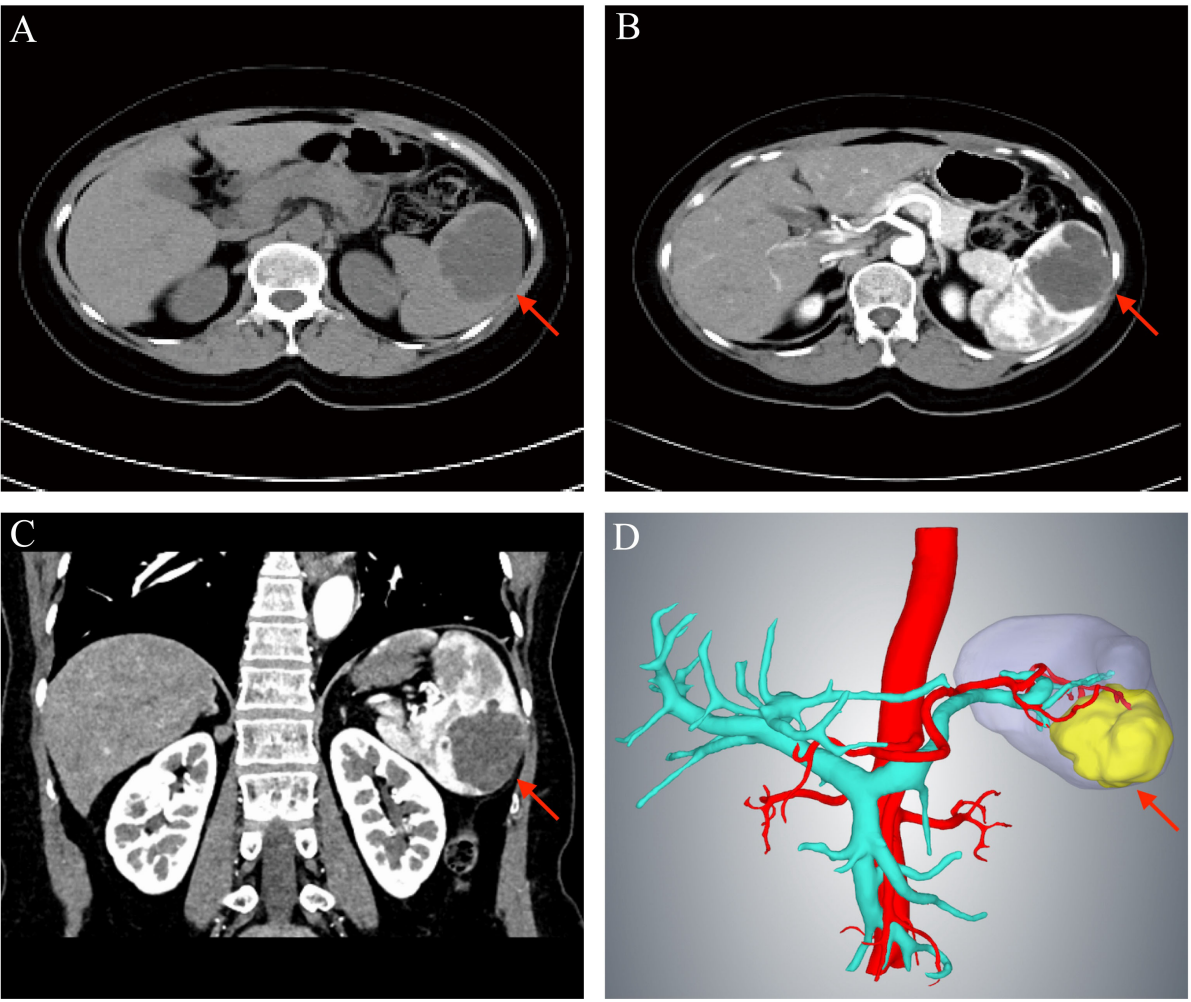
Preoperative examination of the patient. (A) Computed tomography scan showing a mass of about 61×15 mm in the lower pole of the spleen (red arrow). (B) Enhancement of the wall of the capsule with no obvious enhancement within the capsule is observed (red arrow). (C) The red arrow indicated the tumor. (D) Three-dimensional imaging showing the tumor confined to the lower pole of the spleen (red arrow) and supplied by the lower pole splenic artery.
